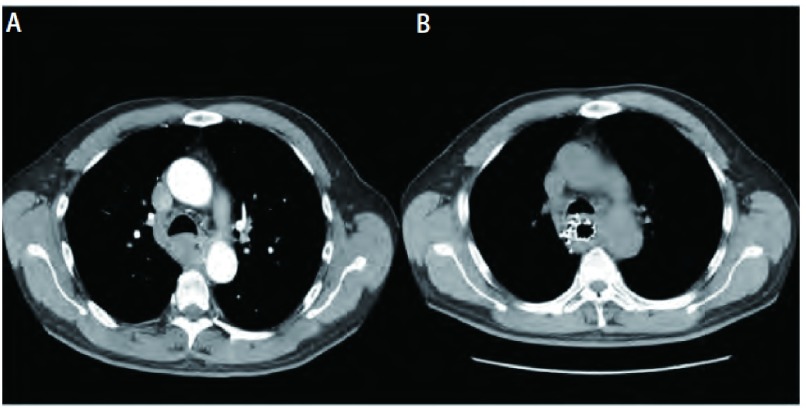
8区食管鳞癌。A:治疗前CT增强扫描显示肿瘤; B:治疗后4个月复查肿瘤NC。 Esophageal squamous cell carcinoma in the eighth region.A:Enhanced CT scanning shows the tumor before therapy; B:4 months after radioactive seeds implantation, tumor outcome is NC.NC:No change.
